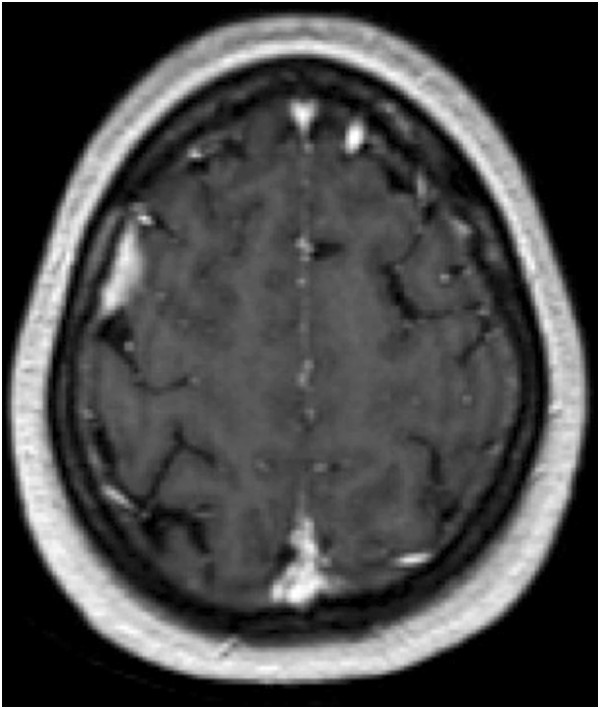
Magnetic resonance imaging with contrast shows enhancement of the meninges in the frontal, parietal and occipital lobes consistent with carcinomatous meningitis.
